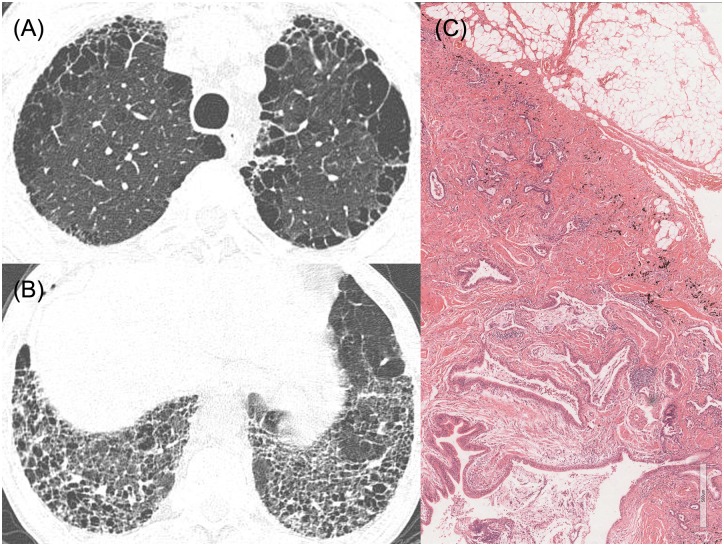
HRCT images and pathologic features of a 64-year-old man who have possible UIP pattern with emphysema. (A), (B) HRCT shows upper lobe-predominant paraseptal emphysema and symmetric honeycombing in lower lobes. (C) Pathologic features show dense fibrosis with severe architectural distortion (x40). He was diagnosed with IPF after multidisciplinary discussion between pulmonologists, radiologists, and pathologists, and the pSRIF score was -3.
